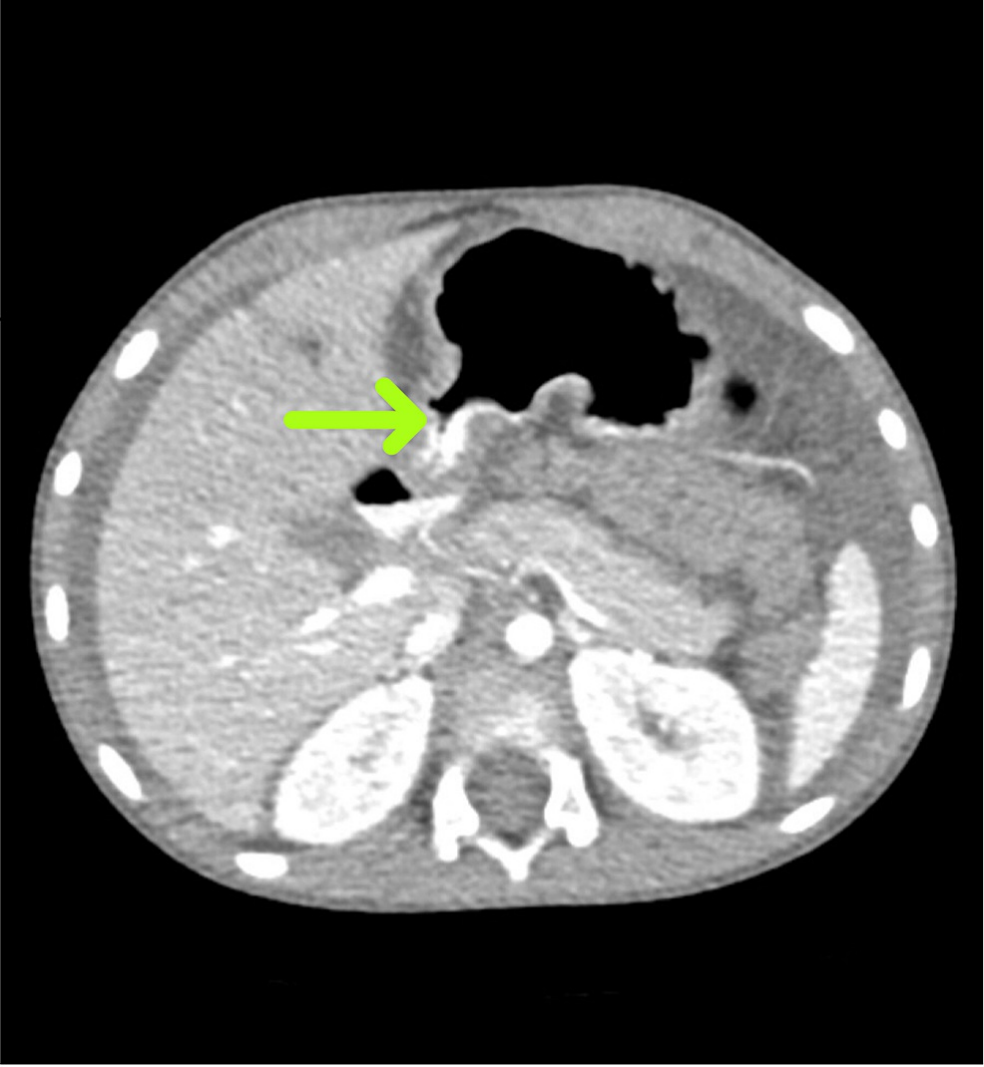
CT scan of the abdomen and pelvis showing possible area where internal hernia was causing obstruction of small bowel loops. Findings were unclear. CT: computed tomography
